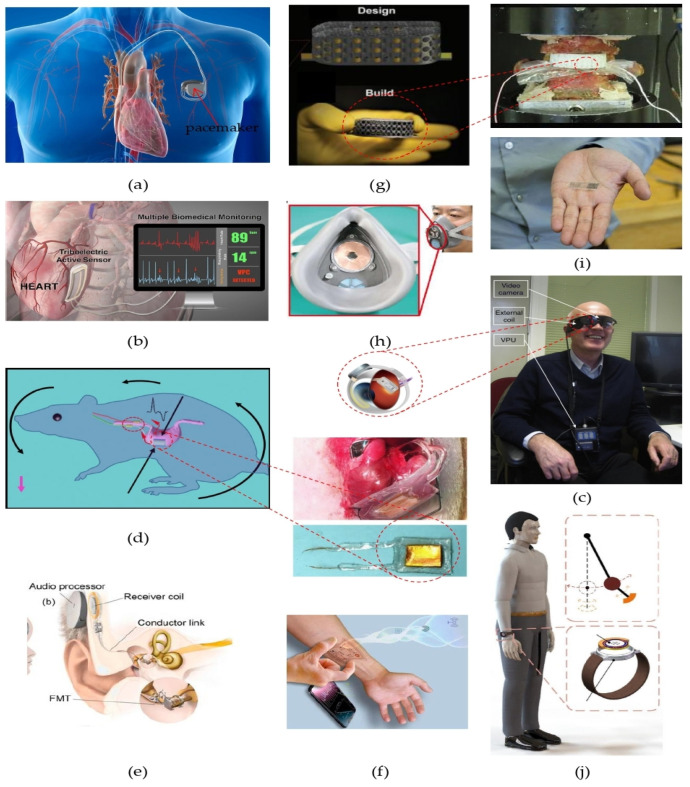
Examples of miniaturized biomedical devices and self-powered implants. Self-rechargeable cardiac pacemaker (a) [72]; troboelectric active sensor (b) [72,73]; retinal prosthesis system, a variable external unit with camera attached to it (c) [74]; self-powered vagus nerve stimulator device for effective weight control (d) [75]; an ultrasonic energy harvester in use in a cochlear hearing aid (e) [76]; energy harvesting from radio waves for powering wearable devices (f) [77]; self-powered metamaterial implant for the detection of bone healing progress (g) [13]; self-powered electrostatic adsorption face mask based on a triboelectric nanogenerator (h) [78]; self-powered implantable device for stimulating fast bone healing, which then disappears without a trace (i); self-powered smart watch and wristband enabled by an embedded generator (j) [79]. Reproduced with permission from [13,72,73,74,75,76,77,78,79].
